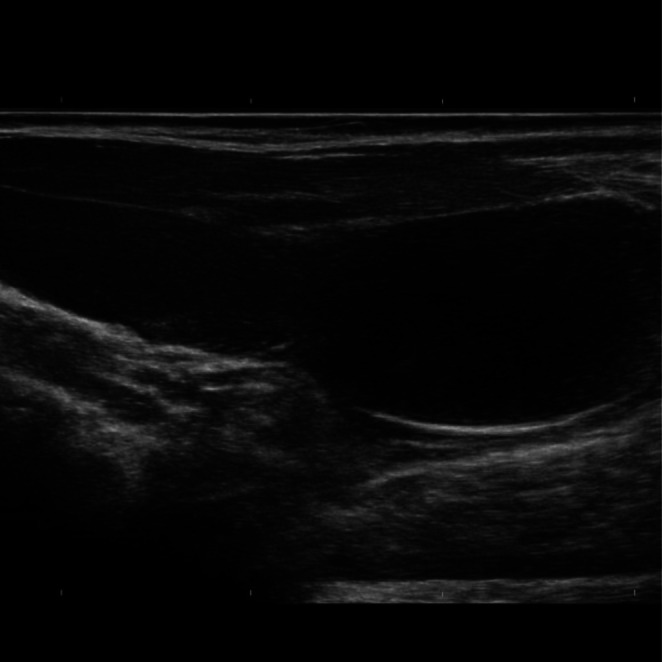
Ultrasonography of the left inguinal region. The left testis was not visualized within the scrotum.
